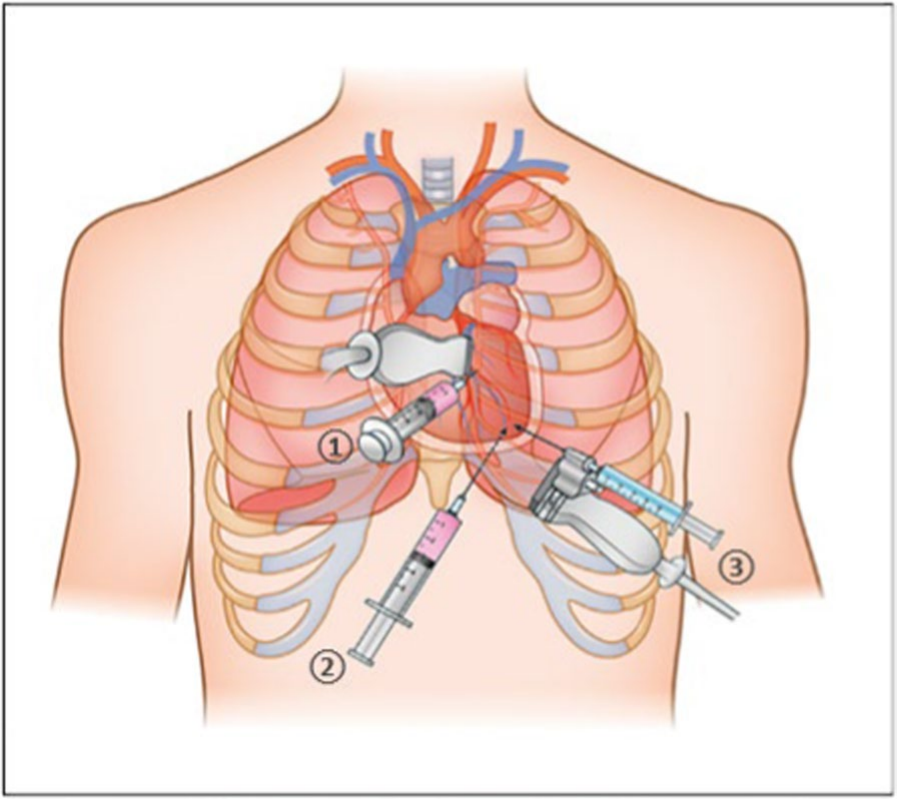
Percutaneous pericardiocentesis approaches; ① parasternal approach, ② subxiphoid approach and ③ apical approach [10]
